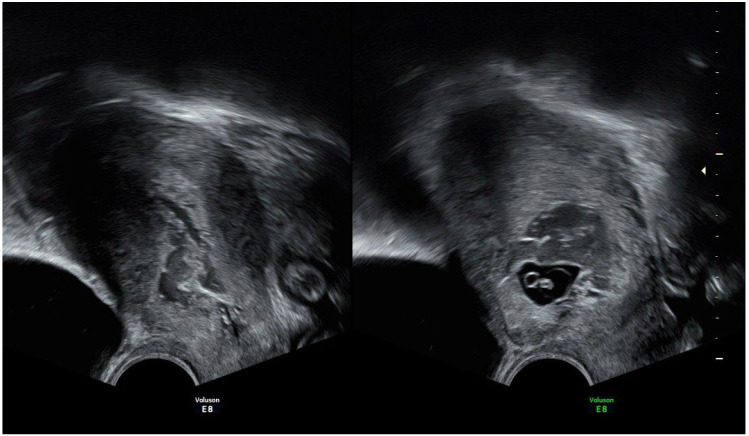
Ultrasound images of the uterus showing a gestational sac in the cesarean scar, with an empty uterine cavity and an empty cervical canal.
